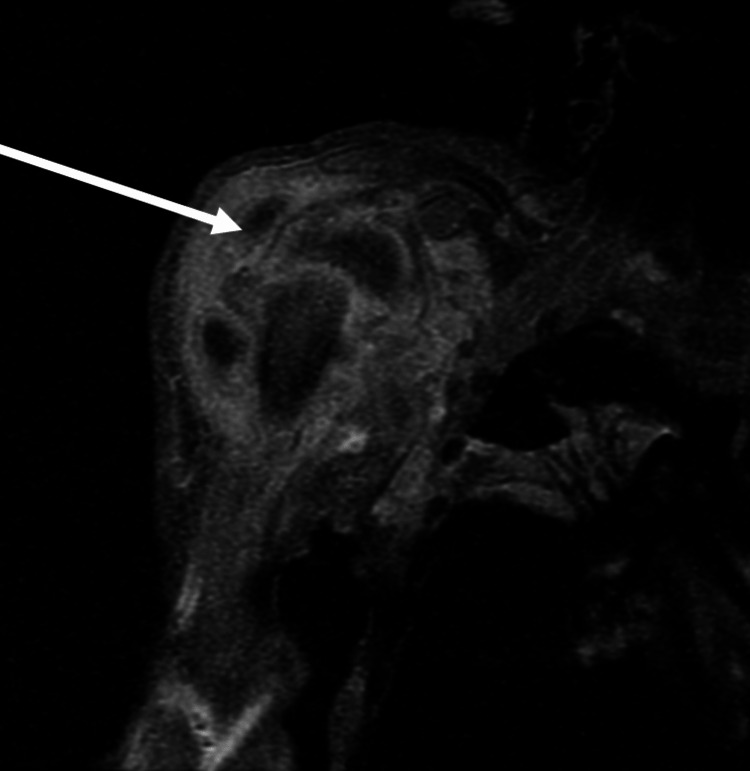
Post-contrast image of MRI right shoulder in Case 2 demonstrated multiple collections in the subacromial subdeltoid bursa with intense surrounding bone marrow edema (long arrow).
